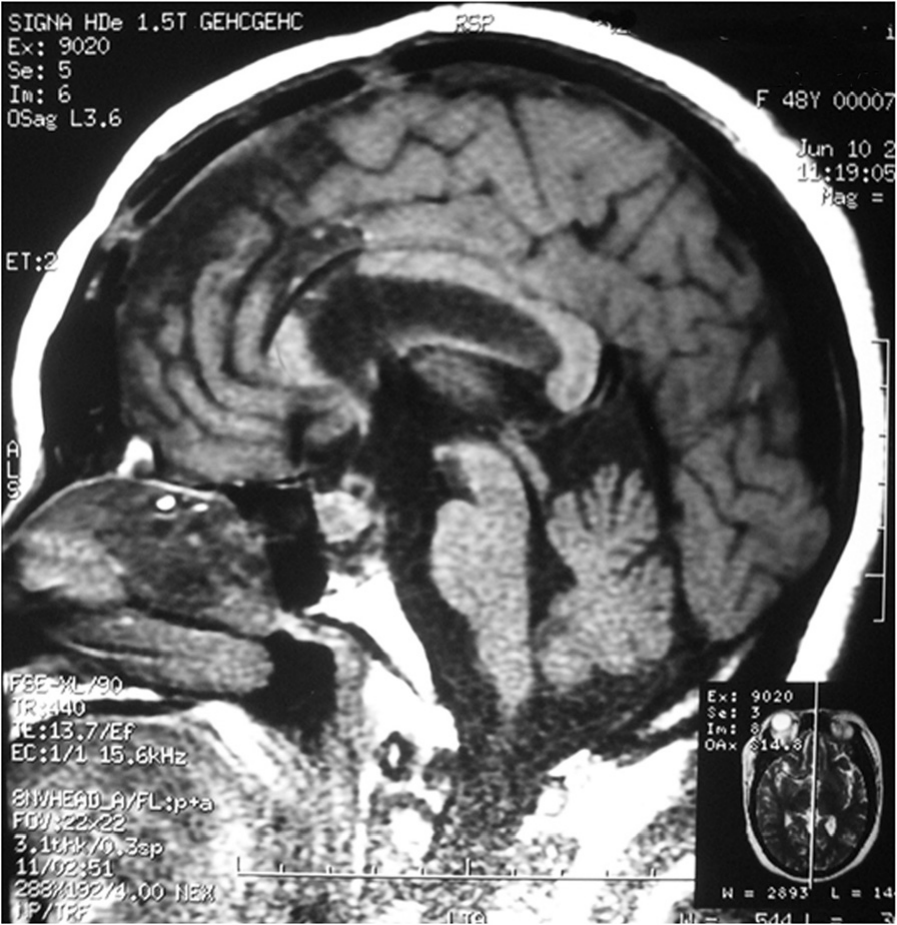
Sagittal T1-weighted MRI scan obtained 3 months after second surgery, revealing total tumor resection.
